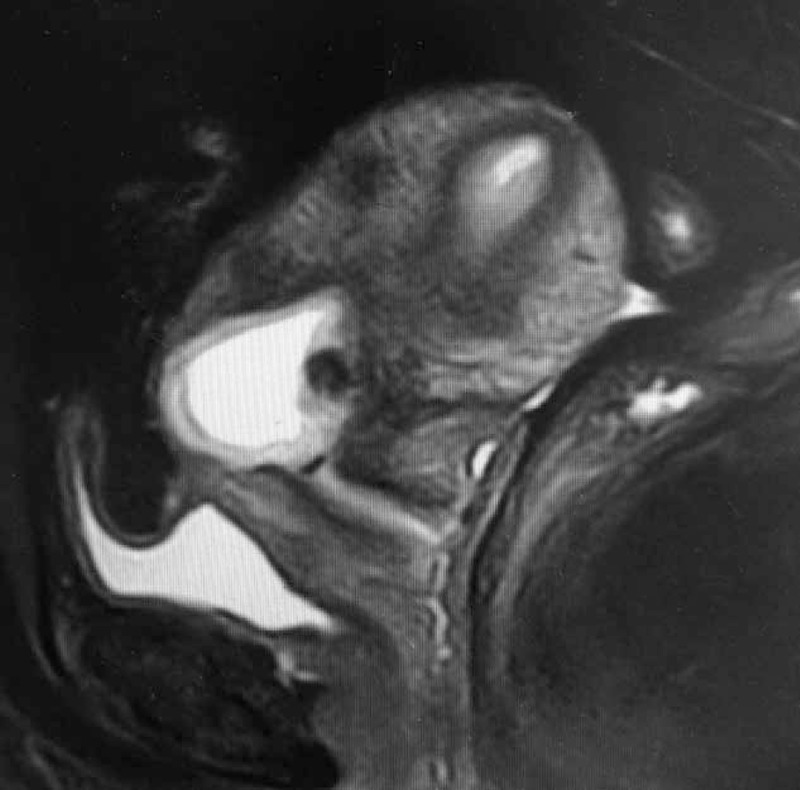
A gestational sac implanted in the previous cesarean scar (sagittal view of the magnetic resonance imaging).
